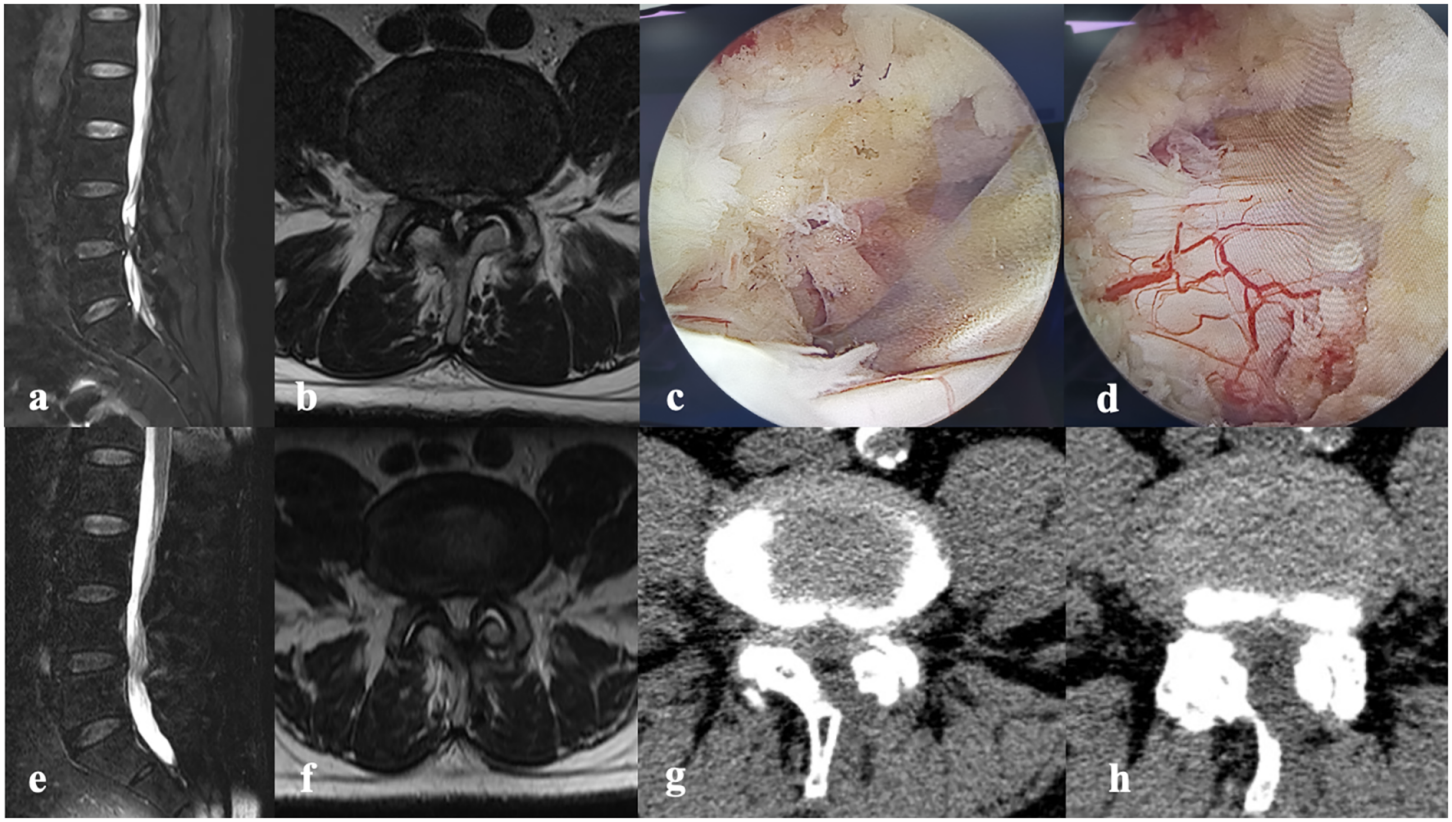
A 72-year-old male had numbness in both lower limbs accompanied by intermittent claudication for 2 years, with symptoms occurring after walking 200 m. No significant positive findings on physical examination. ODI score is 40. (a) The sagittal view of MRI. (b) The axial of MRI. (c,d) Relieved compression under endoscopy. (e,f) The sagittal/axial view of MRI showing the lumbar spine after surgery. (g,h) The axial view of CT.
